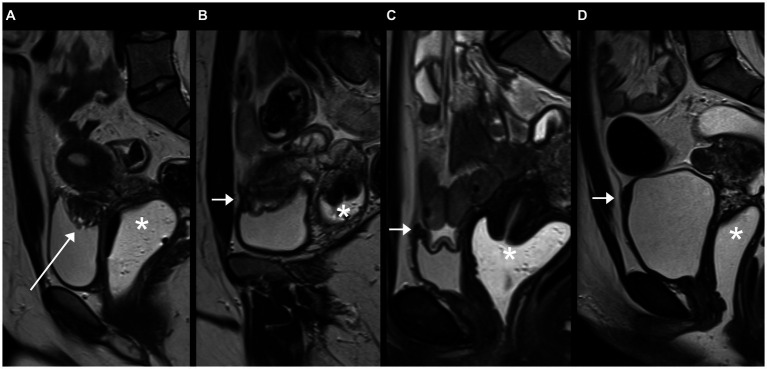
Sagittal T2 FSE (fast spin echo) slices demonstrating bladder findings of different patients on MRI: (A) Typical deep infiltrating endometriosis (DIE) of the bladder dome (hypointense mass with hyperintense foci, long arrow), rated #Enzian FB by all three readers, (B) focal thickening of the anterior bladder wall (short arrow), scored #Enzian FB by one of three readers due to off-midline location and central T2w-hyperintensity (no endometriosis on laparoscopy), (C) and (D) focal thickening of the anterior bladder wall (short arrows), interpreted as prominent urachal remnant by all three readers due to midline location on the serosal surface and the presence of a thin band, extending from the bladder dome toward the umbilicus. Asterisks: vaginal vault.
